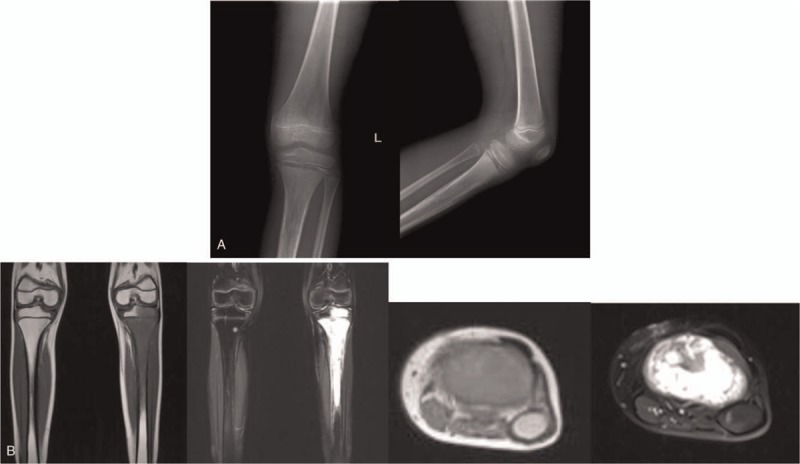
(A) The X-ray of knee showed bone mineral density reduction of left upper middle tibia, Local bone a periosteal reaction can be seen. (B) The MRI of knee showed an abnormal signal of the left upper middle tibia, metaphysis, and epiphysis. The lesion showed hypointensity on T1-weighted imaging and hyperintensity on T2-weighted imaging. Surrounding soft tissue was swelling with a 3 cm × 3 cm mass. MRI = magnetic resonance imaging.
